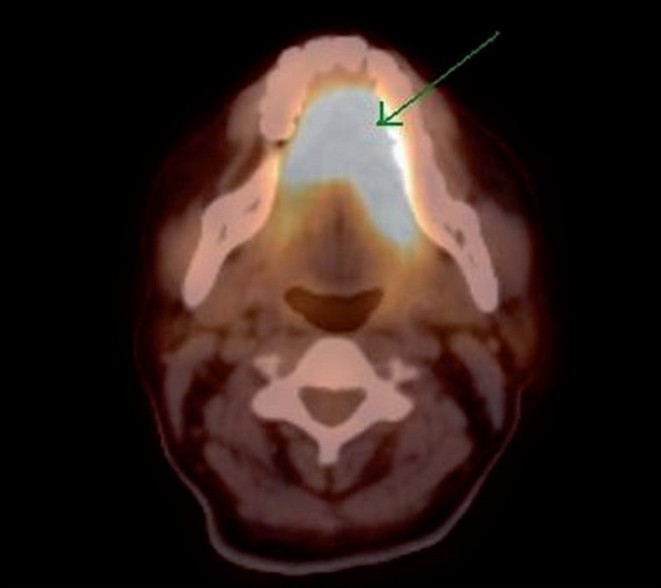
Transaxial section of the positron emission tomography-computed tomography fusion image showing intense fluorodeoxyglucose uptake in the anatomically normal tongue
